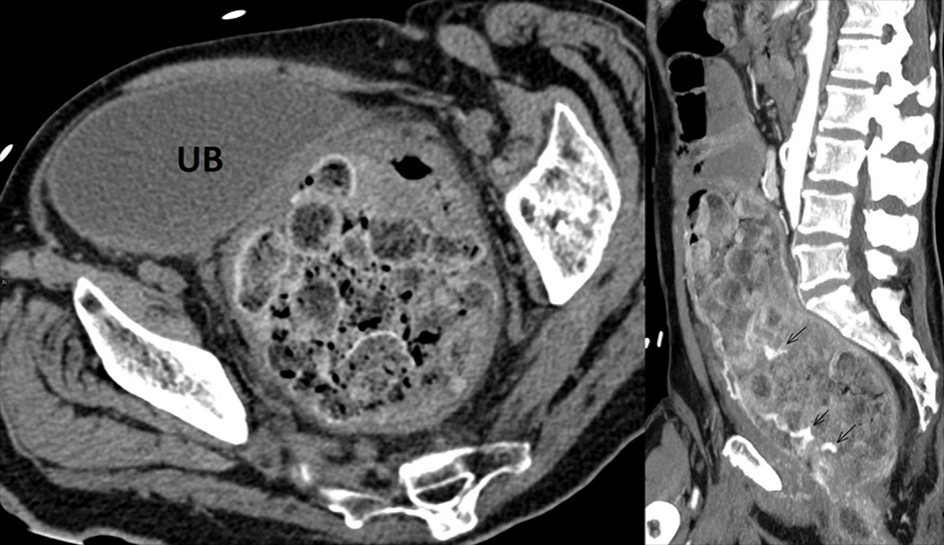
On the left, the axial unenhanced CT image shows the dilated rectum filled with numerous stercoral stones and causing extrinsic compression on the UB. On the right, a sagittal maximum intensity projection reconstruction of the arterial enhanced scan shows the true extent of the faecaloma and the intraluminal contrast material between the stercoral stones (arrows). UB, urinary bladder.
